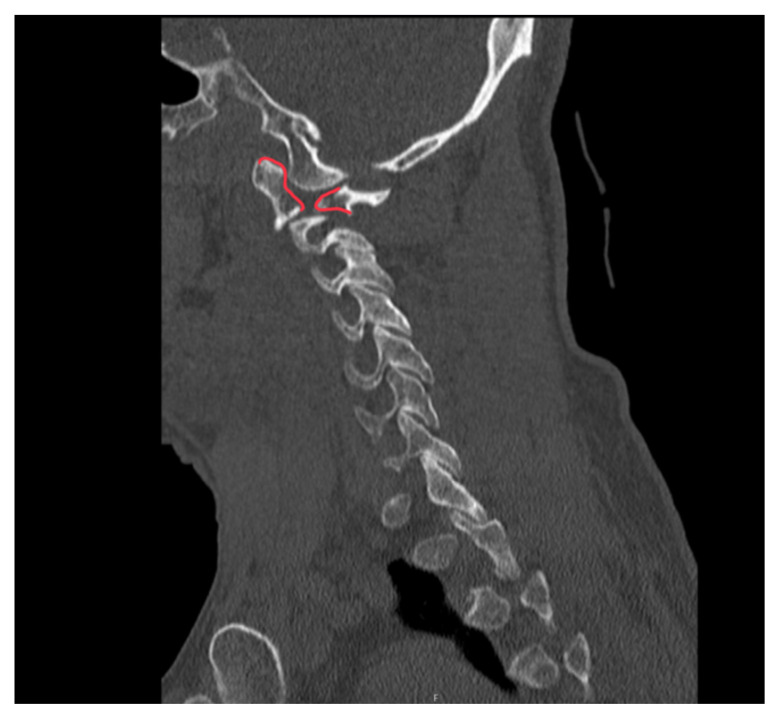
Sagittal CT Video Link: https://youtu.be/m-PII5fD29c
